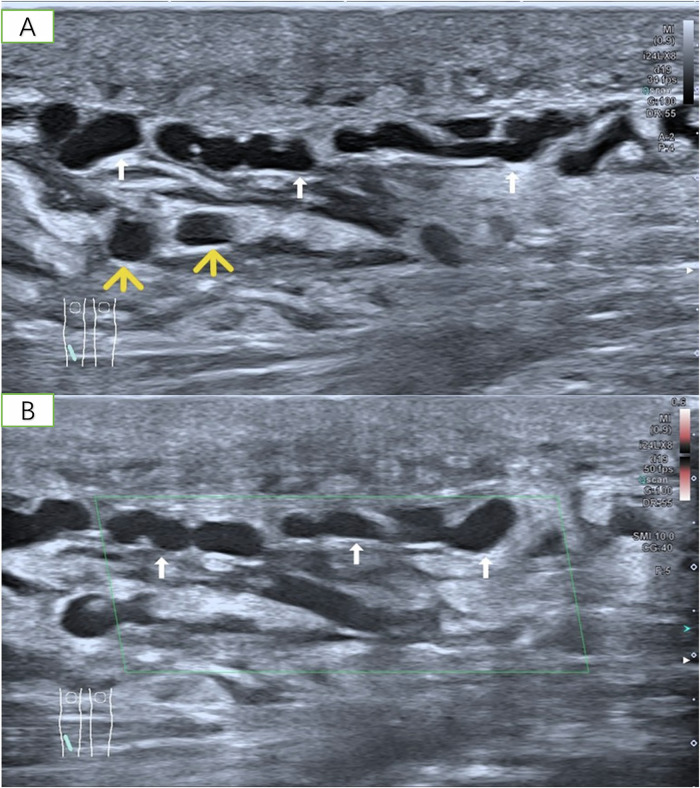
A HFUS indicates lymphatic vessel (yellow arrow) is a structure with a circumferential annular hyperechoic and central anechoic structure on the transverse section, and parallel hyperechoic linear wall structures accompanied by anechoic lumens in the longitudinal section (white arrow). B SMI indicates no obvious blood flow signal in the lumen of the lymphatic vessel. HFUS, high-frequency ultrasound; SMI, Superb Micro-vascular imaging
